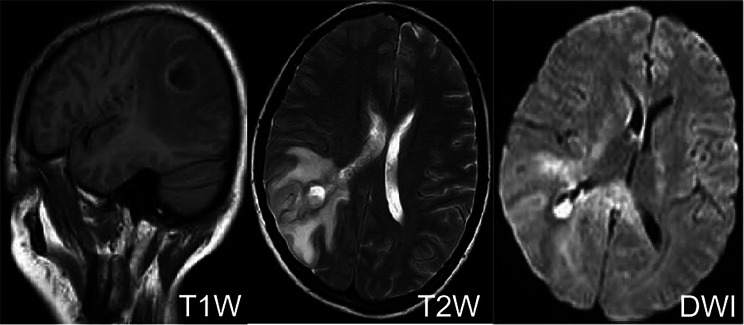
Magnetic resonance images of the brain abscess. TIW = T1-weighted; T2W = T2-weighted; DWI = diffusion weighed imaging. A patchy abnormal signal was observed in the right parietal–temporal lobe, with a low signal on T1-weighted imaging surrounded by annular high signals, and a high signal on T2-weighted imaging surrounded by annular low signals around the lesion. Diffusion-weighted imaging showed high signals with patchy long T1 and long T2 signals around the lesion; the lesion appeared to be connected to the right lateral ventricle
